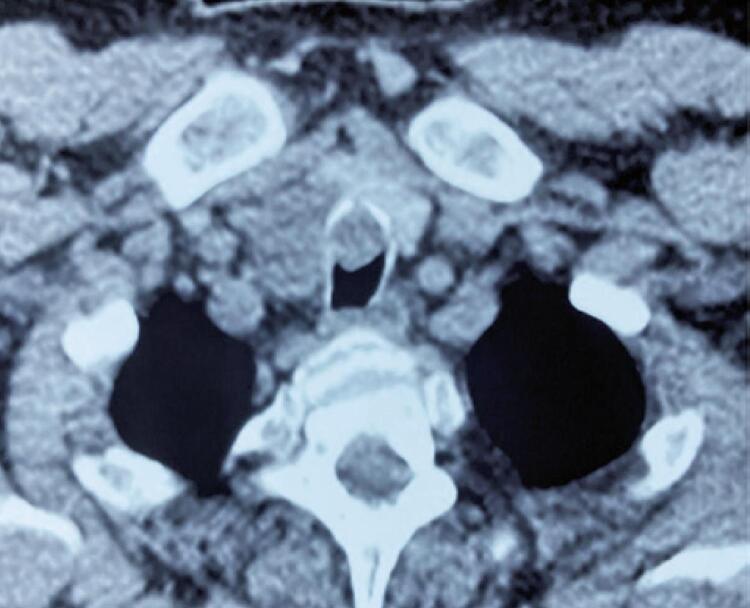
Computed tomography of the neck with contrast showing evidencing cervical tumor with extension to tracheal lumen
